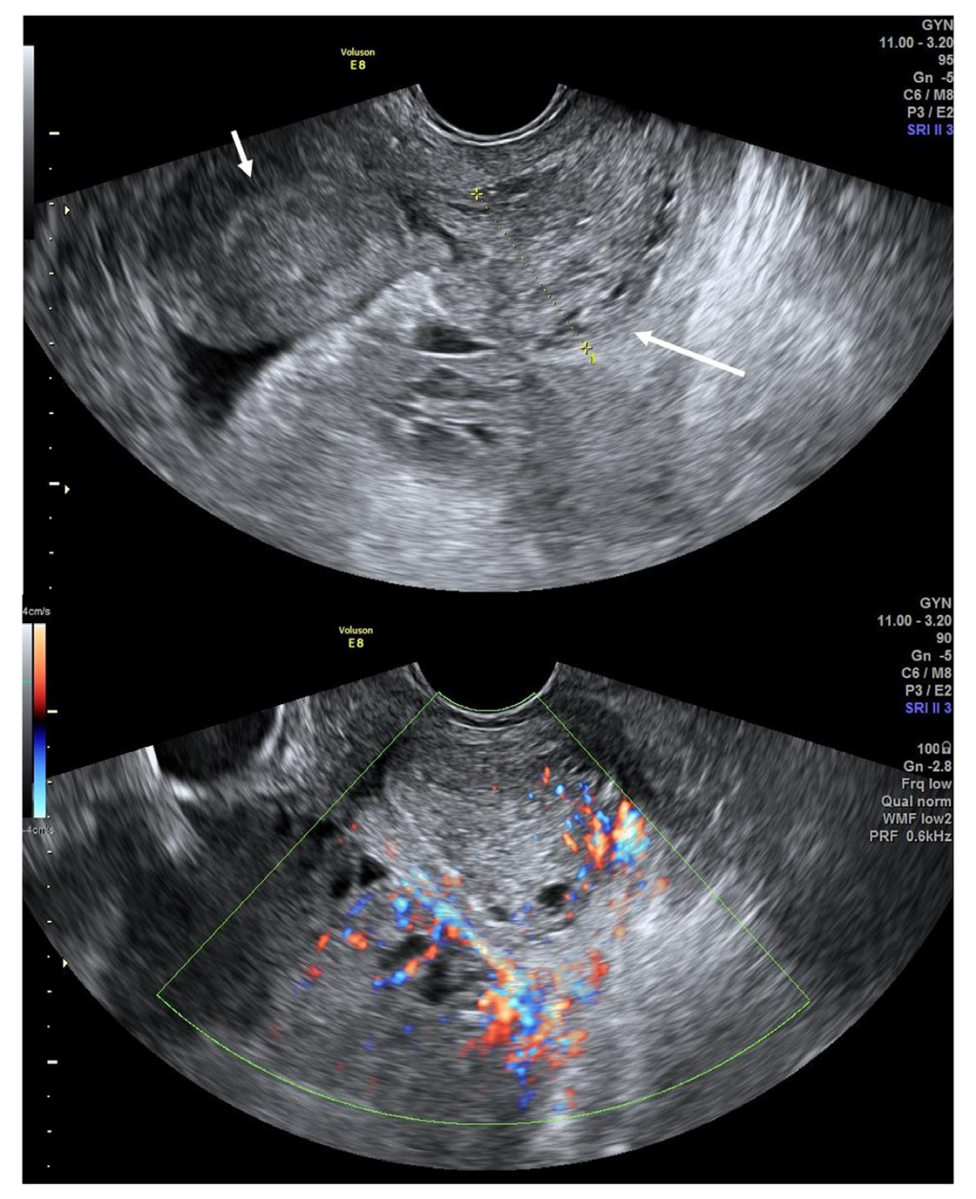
Transrectal ultrasonography. Ultrasonography shows a 1.5-cm-thick endometrial layer in the right uterus (short arrow) and up to 2.69 cm in the left uterus (long arrow). The mass was connected to the left uterus and appeared to be extending into the vaginal cavity. Internal blood flow was increased.
